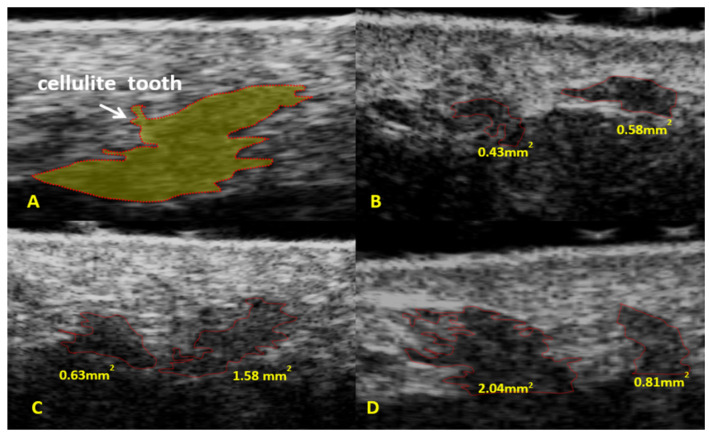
Ultrasound assessment of fat protrusions at the dermal subcutaneous junction (A). Surface area of fat protrusions at the dermal subcutaneous junction: (B)—group 1; (C)—group 2; and (D)—group 3.
